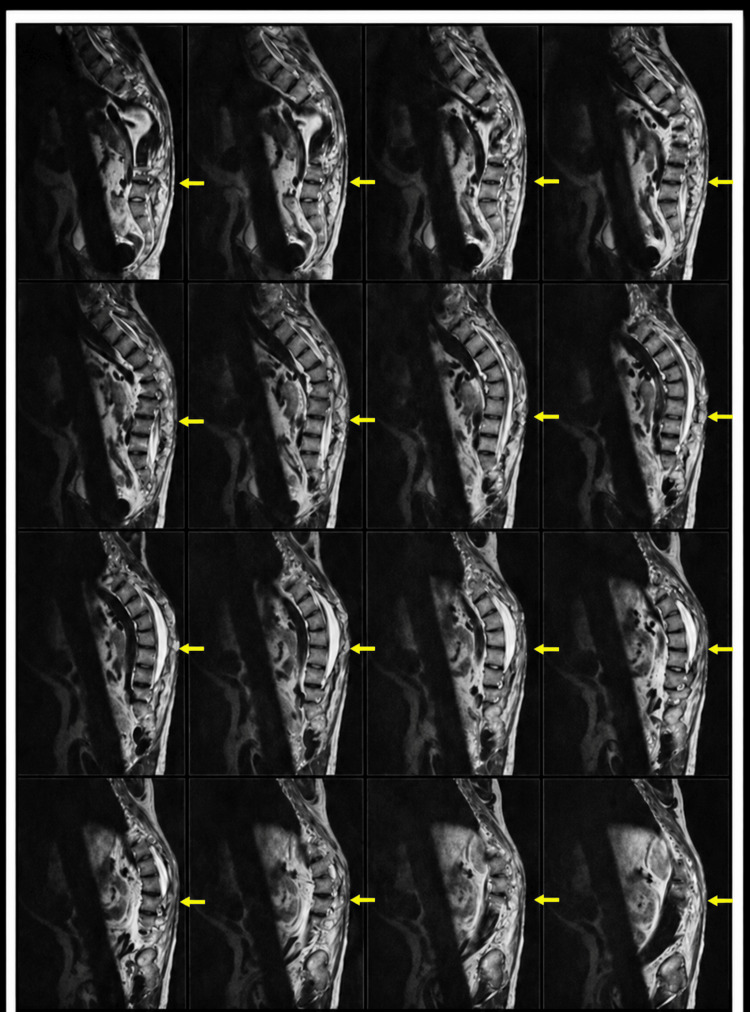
Sagittal CT images of the spine demonstrating extensive ankylosis with fusion of the posterior vertebral elements in advanced ankylosing spondylitis (arrows).
